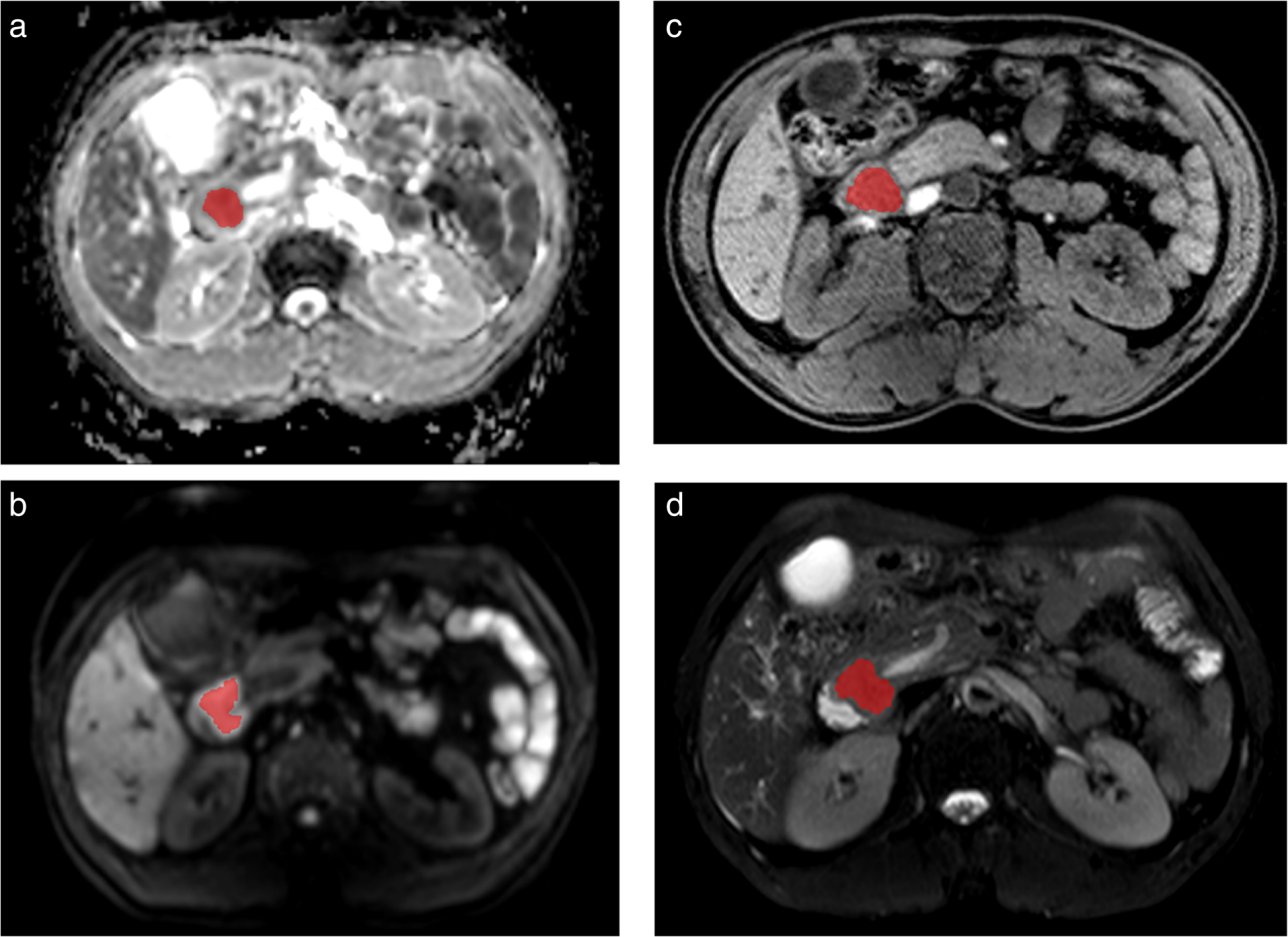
ROI was placed on the maximum section of the tumor, avoiding adjacent vessels and bile duct on ADC (a), DWI (b), T1WI (c), and T2WI (d), respectively
